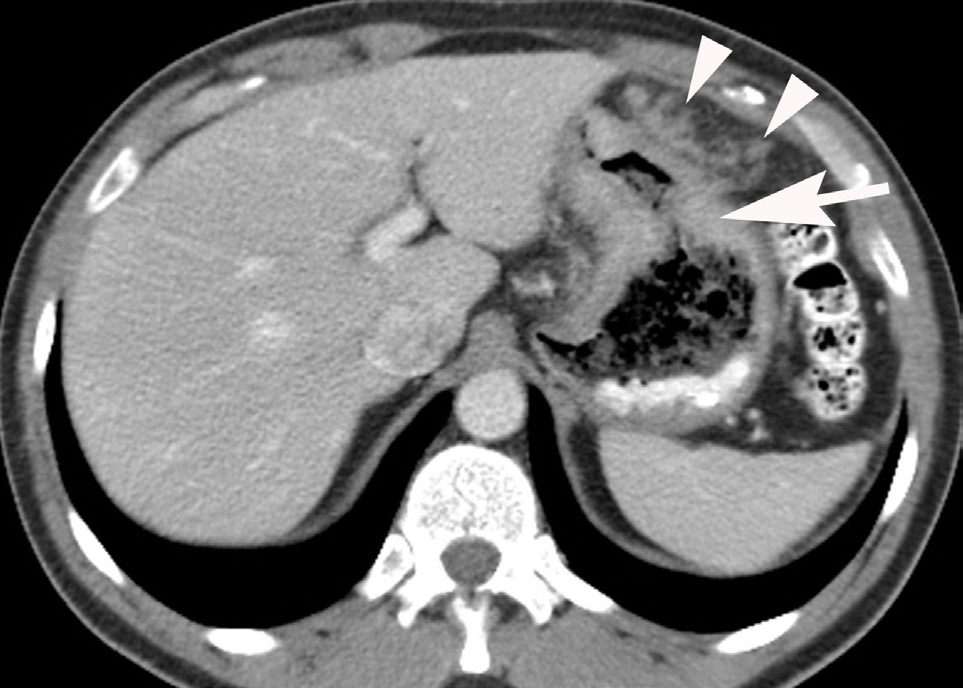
Intravenous and oral contrast material-enhanced computed tomography (CT) showing an adenocarcinoma producing irregular wall thickening of the proximal body of the stomach (arrow) with adjacent omental spread of tumor (arrowheads).
